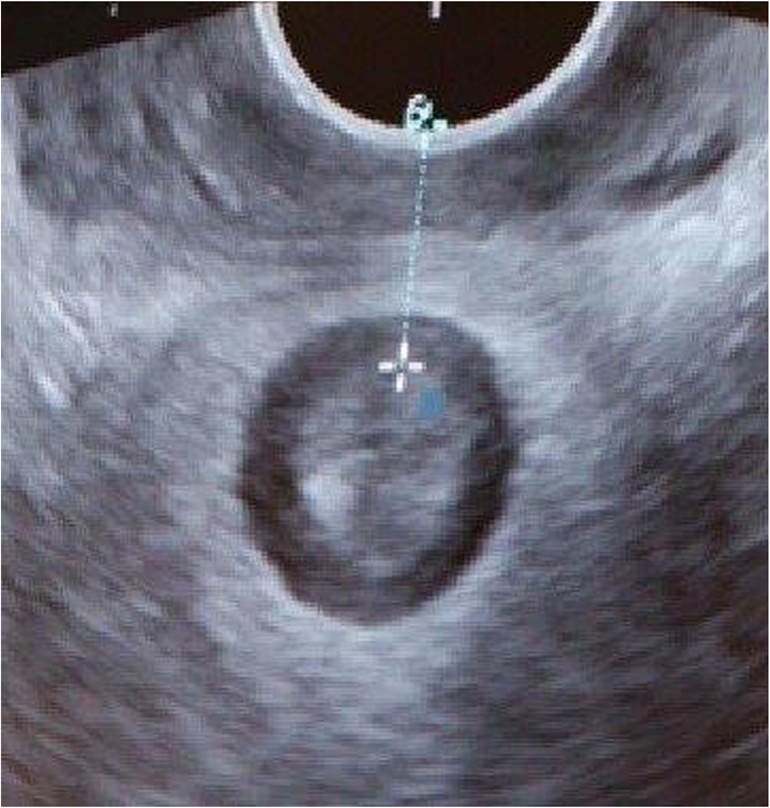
Perineal ultrasound image of the anovaginal distance measured from the anal mucosa to the posterior vaginal wall
